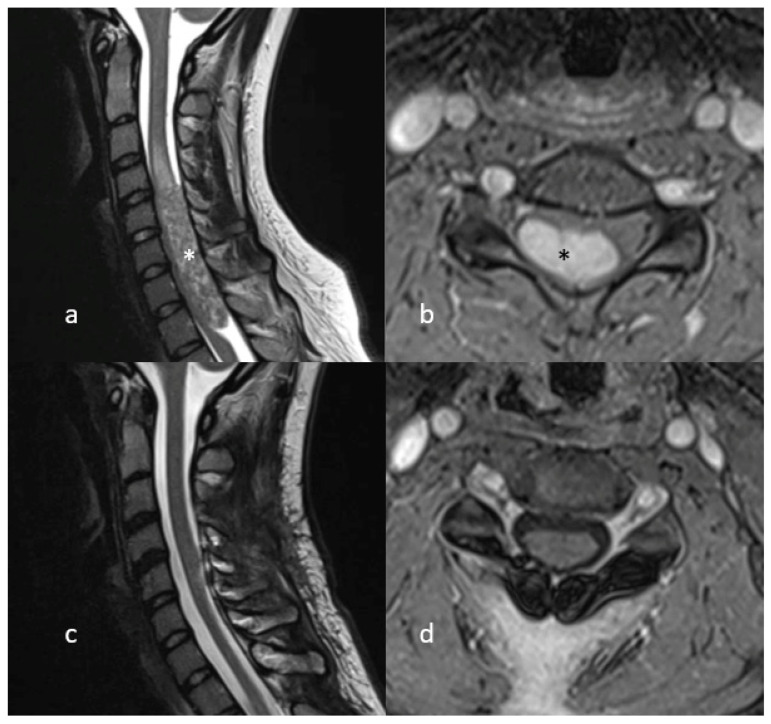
MR Imaging (T2-weighted on the left, T1 with gadolinium on the right) showing a large intradural, contrast-enhancing lesion (marked with asterisk) compressing the cervical spinal cord (a,b). Postoperative MR Imaging showing no signs of tumour recurrence (c,d).
